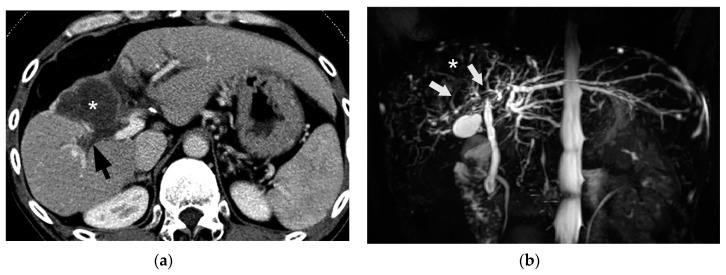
Comparison between CT and MR cholangiopancreatography (MRCP) findings of BDN. (a) On contrast-enhanced CT, a hypodense tubular structure is observed in the hilar area of the right liver lobe (arrow). Adjacent to this tubular structure, a large hypodense mass is observed in the medial segment of the liver (*). These structures are BDN (arrow) and a biloma (*). A dilated intrahepatic bile duct is also observed in the lateral segment of the liver (arrowheads). (b) On MRCP, although dilated intrahepatic bile ducts in the left lateral lobe of the liver are clearly observed, bile ducts in the hilar region and right hepatic lobe are unclear (arrow). Furthermore, the biloma in the medial segment of the liver is not clearly depicted on MRCP (*).
